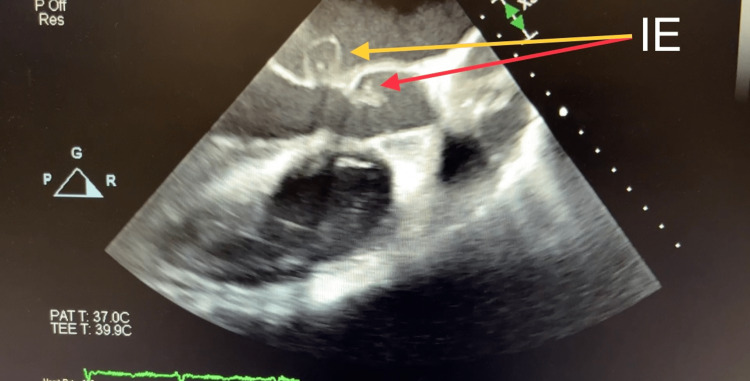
Transesophageal Echocardiogram Showing Infective Endocarditis Involving Aortic Valve and Forming a Fistula in the Aortomitral Curtain
